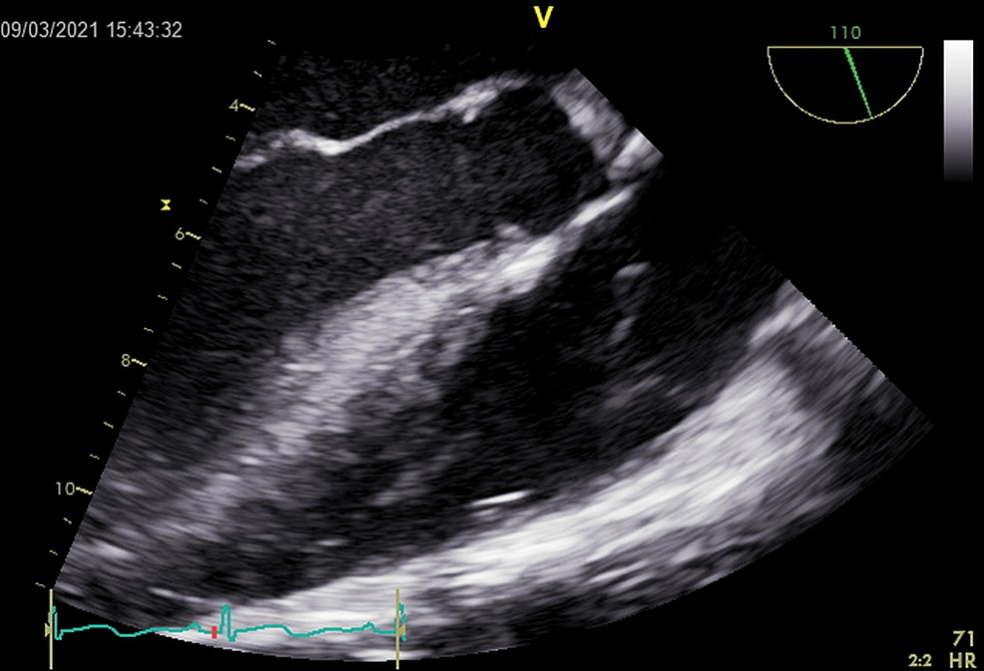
Transesophageal echocardiogram showing 1.1 X 0.5 cm vegetation noted on the pulmonic valve
